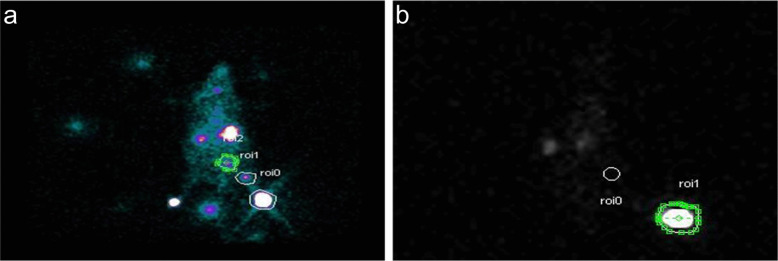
A sample of a positive lymphoscintigraphy and b negative lymphoscintigraphy
